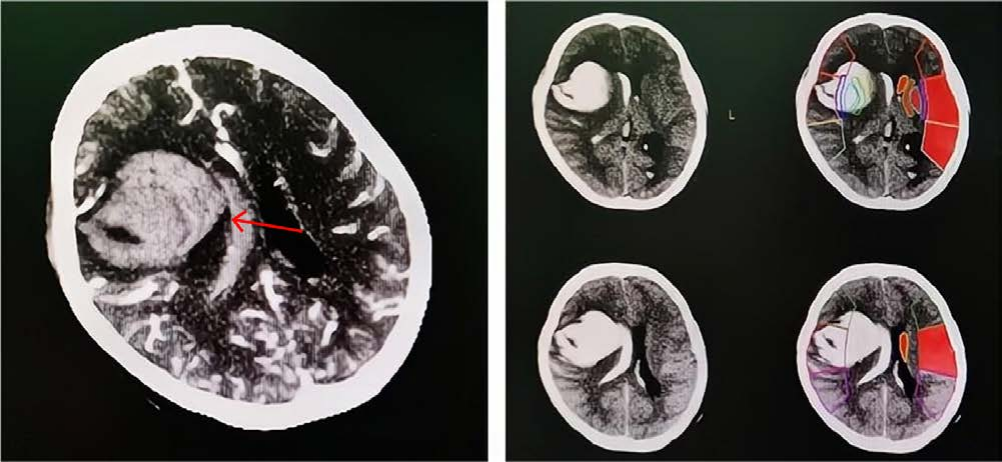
CT of patient’s brain shows the lesion of AVM (arrow). AVM = arteriovenous malformation.
